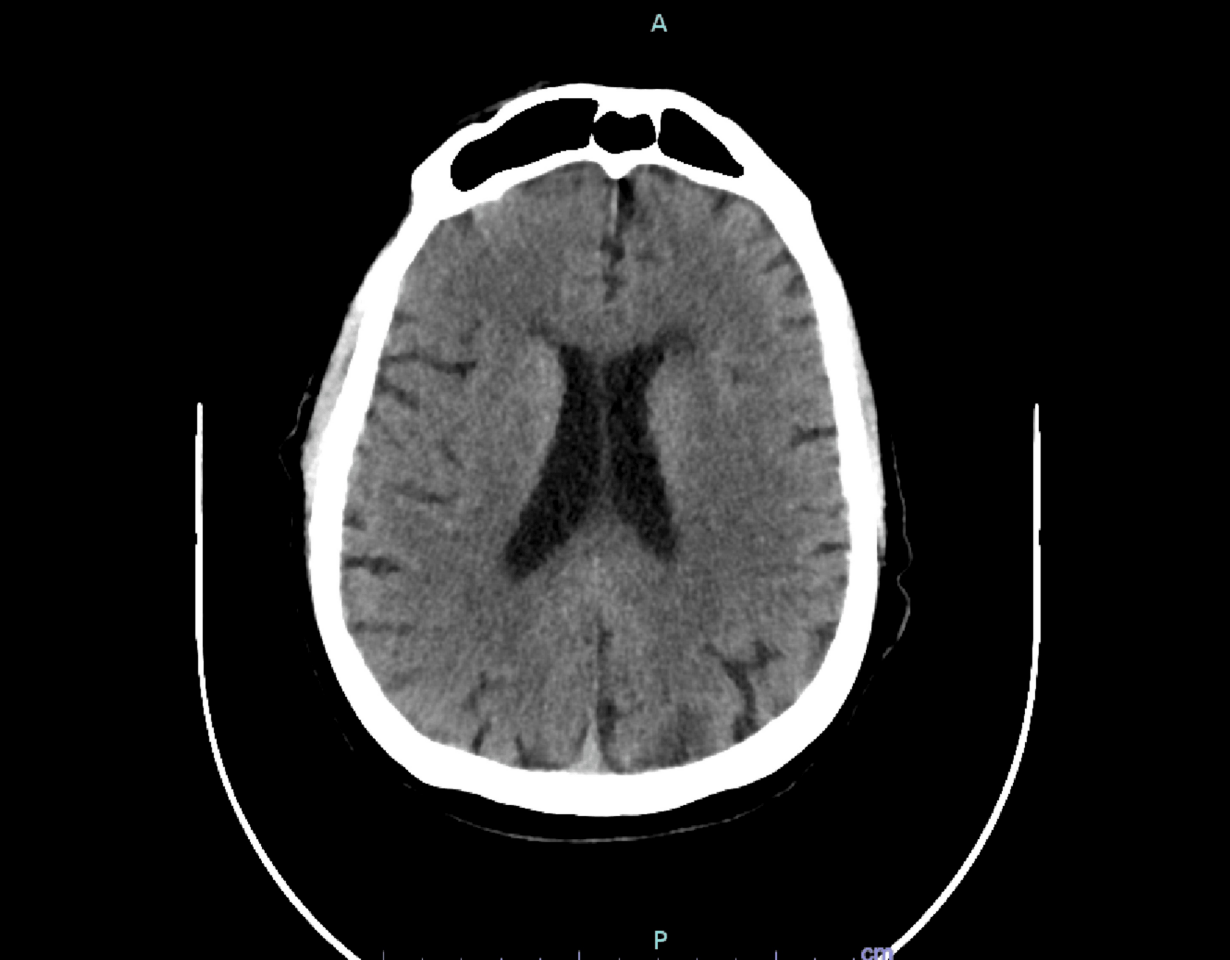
Computed tomography of the head showed no acute abnormalities
